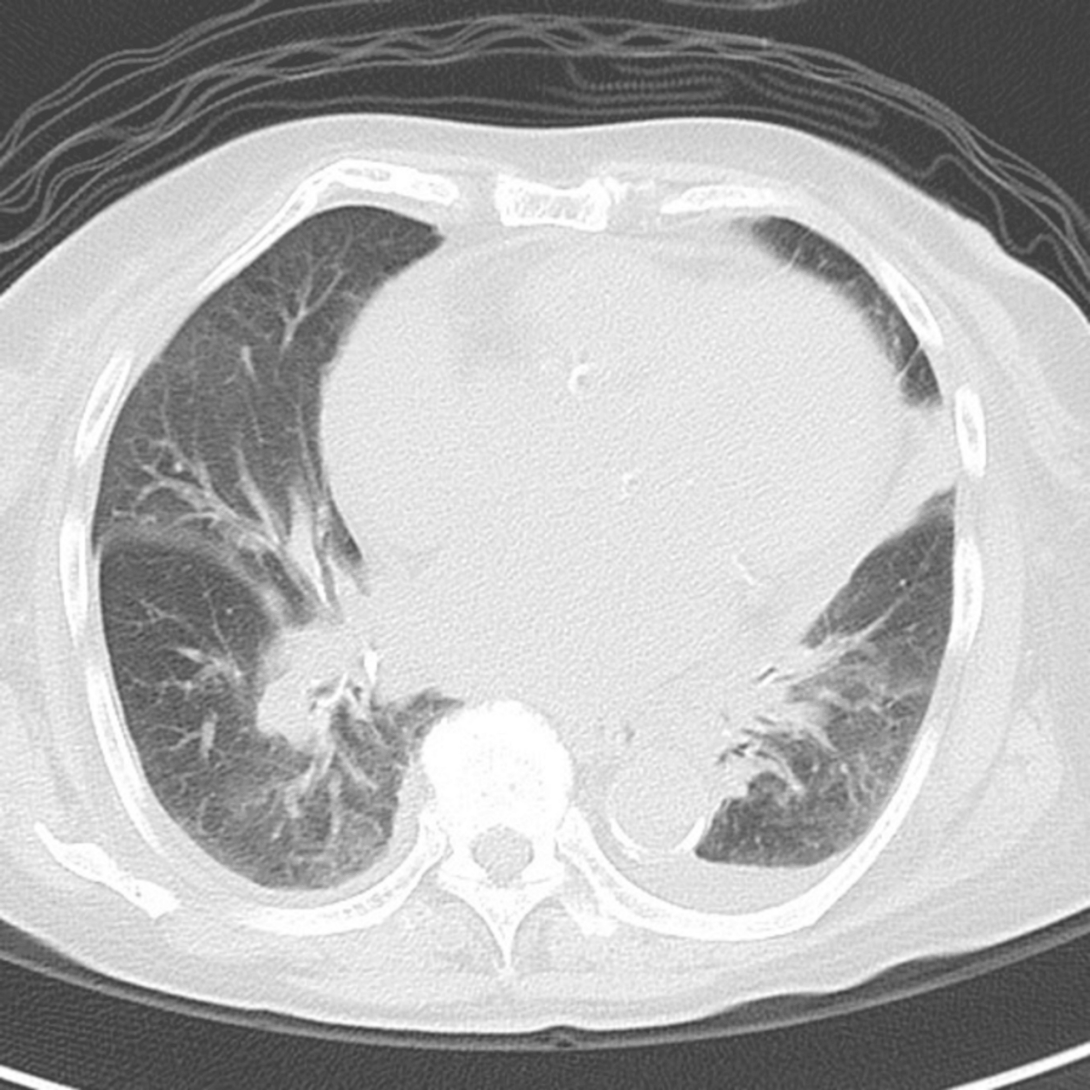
Plain chest computed tomography (CT) Plain chest CT revealed cardiac dilation, pulmonary edema, and mild pleural effusion.
